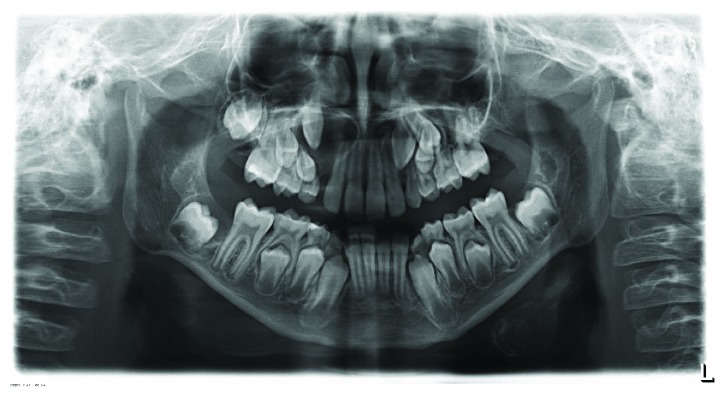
Panoramic radiograph (August 2012). 6 months after therapy, the radiolucent area in the region of tooth 23 was controlled. On the basis of the previous pathological findings, no further surgery was indicated.
